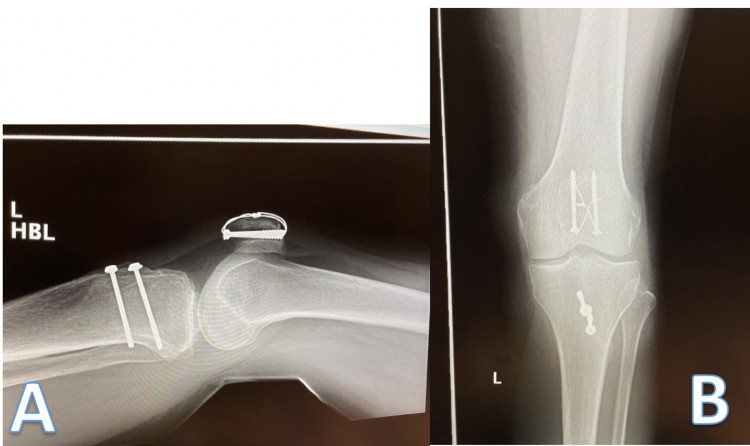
Lateral (A) and anterior-posterior (B) radiographs taken six weeks post-operation.
